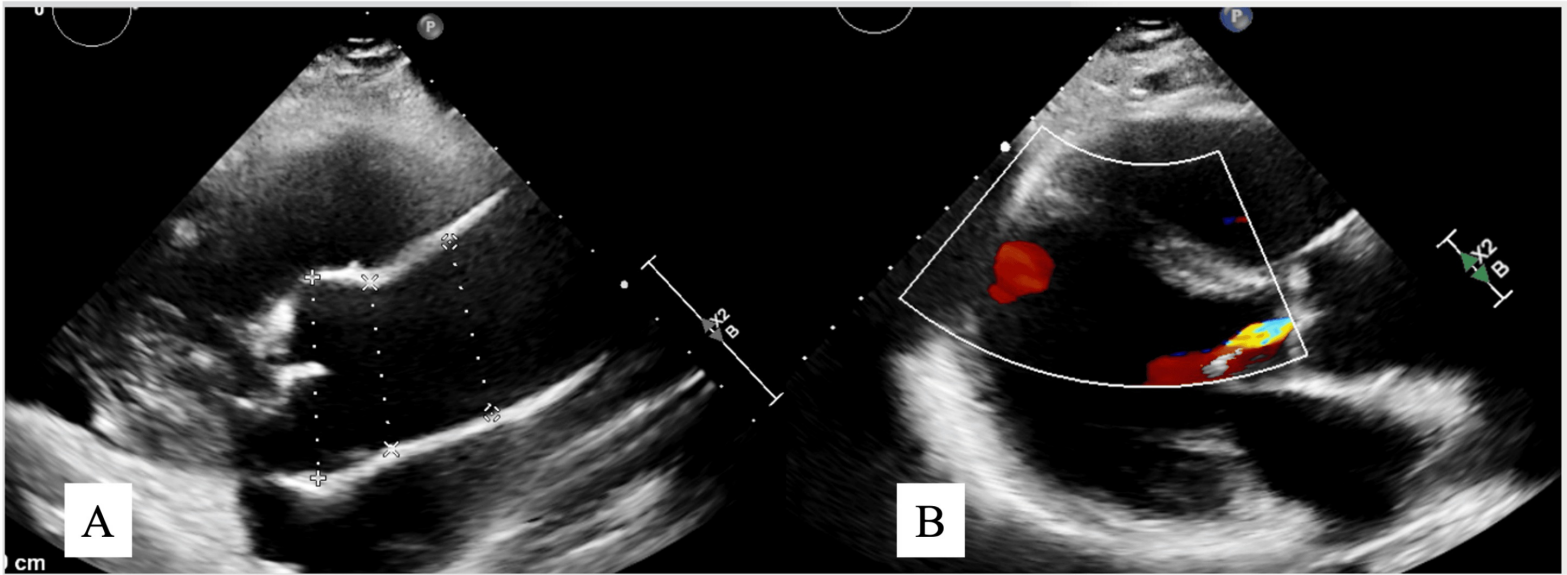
TTE demonstrating left ventricular dysfunction and aortic regurgitation Parasternal long-axis TTE images with two-dimensional measurements (A) color Doppler (B) and demonstrating reduced left ventricular systolic function (LVEF 43%) and moderate-to-severe aortic regurgitation. The left ventricular cavity size is at the upper limits of normal, and the aortic root is mildly dilated (3.9 cm). Mild mitral regurgitation is present, with normal right ventricular size and systolic function and mild pulmonic insufficiency. TTE: transthoracic echocardiography; LVEF: left ventricular ejection fraction
